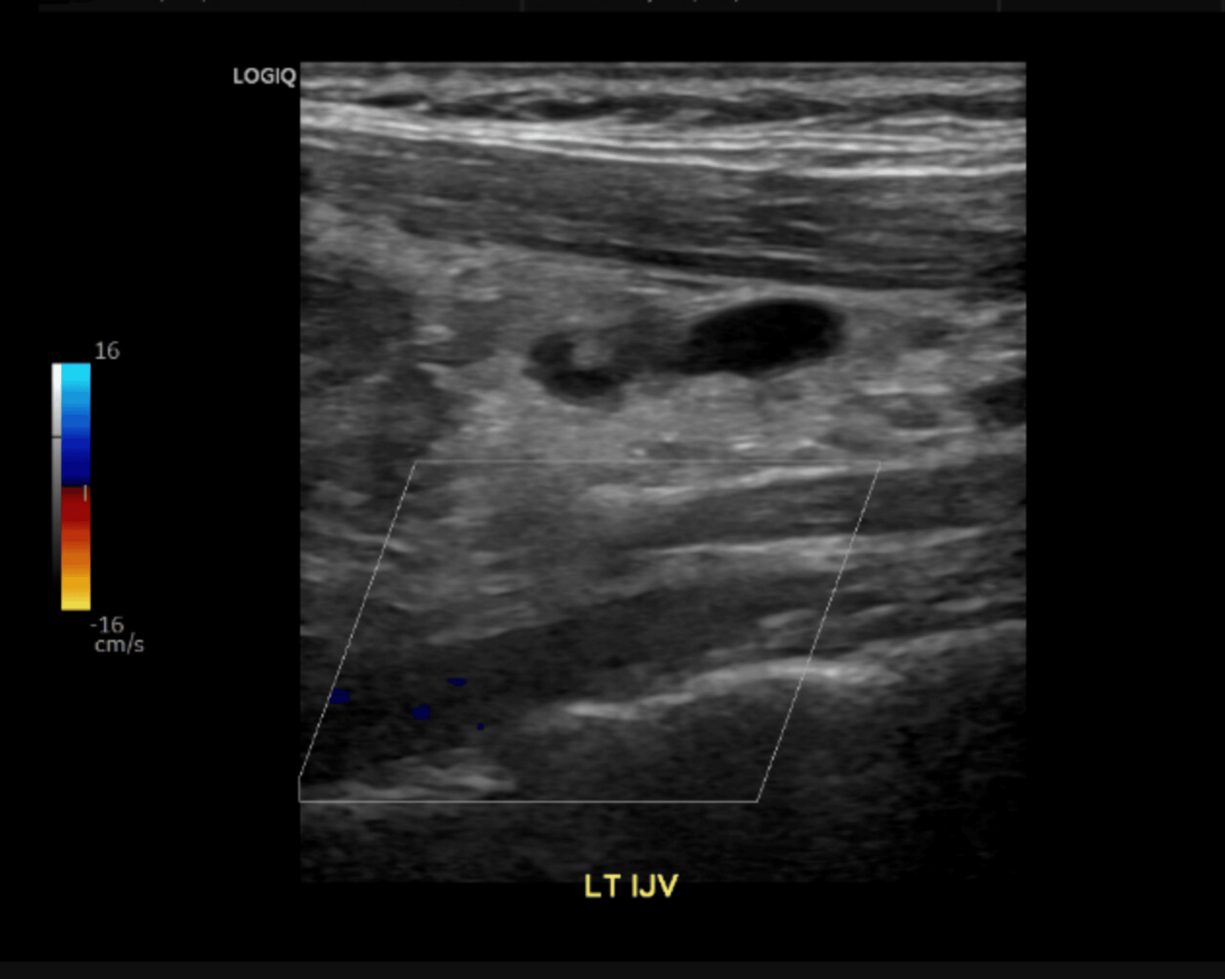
Doppler ultrasound. Upper extremity Doppler ultrasound showing occlusive thrombus in the left internal jugular vein (LT IJV).
